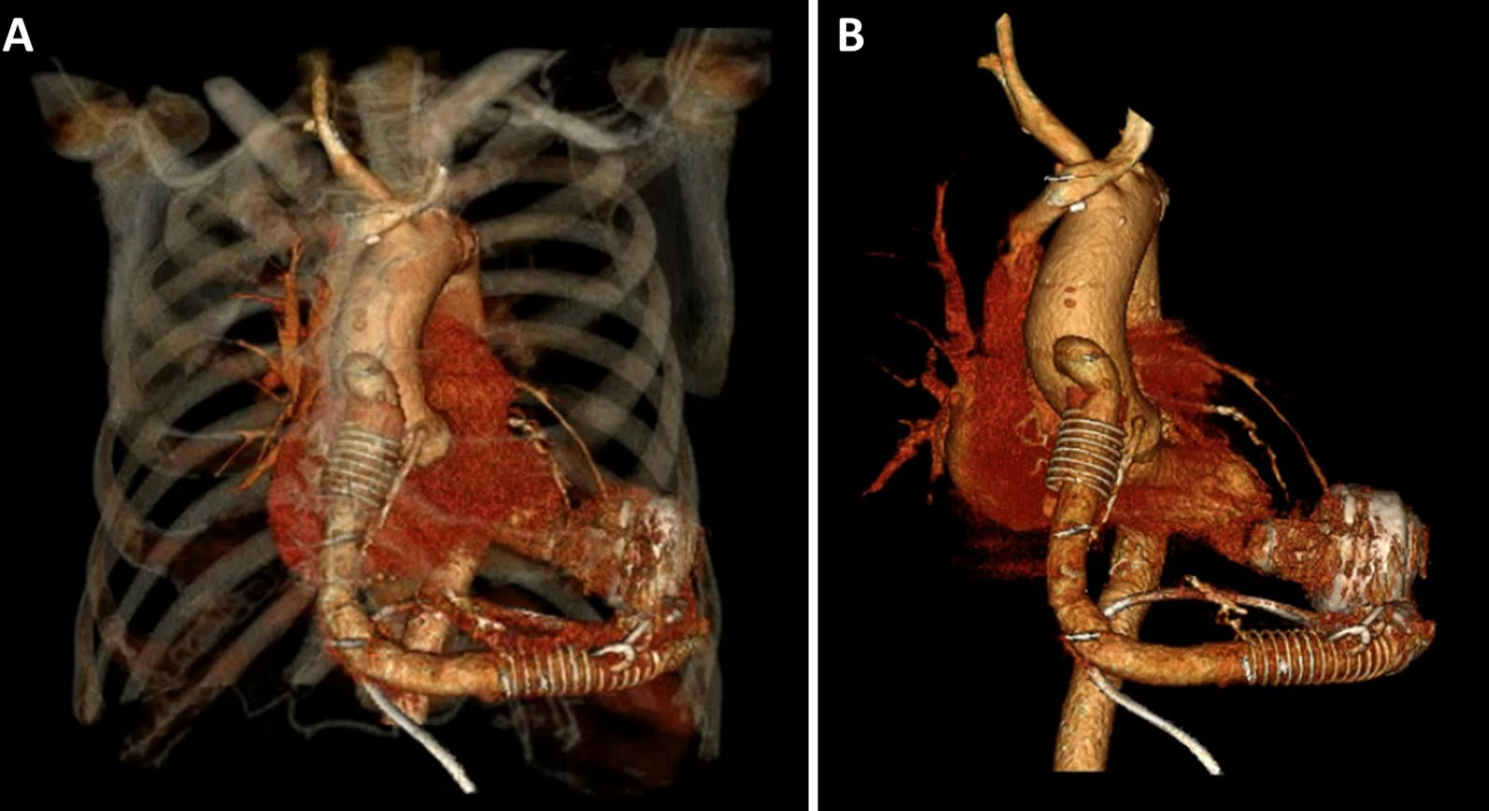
Computed tomography after pump exchange from HMII to HM3
